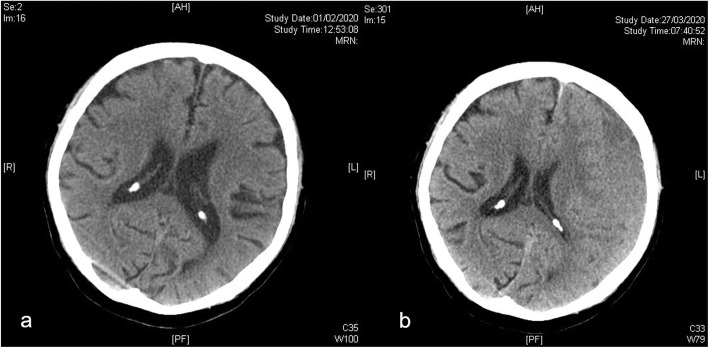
a CT brain scan of an 86-year-old gentleman at the time of injury. He had a minor head injury due to postural hypotension and his anti-hypertensive medication was titrated. CT scan initially showed no intracranial hemorrhage and he was discharged with medical advice. He subsequently had a gradual onset of worsening dizziness. b He presented to the hospital 7 weeks and 5 days after his initial minor head injury. The new CT showed a newly diagnosed left-sided chronic subdural hematoma with mass effect and midline shift. He had a good recovery after emergency burr hole drainage
